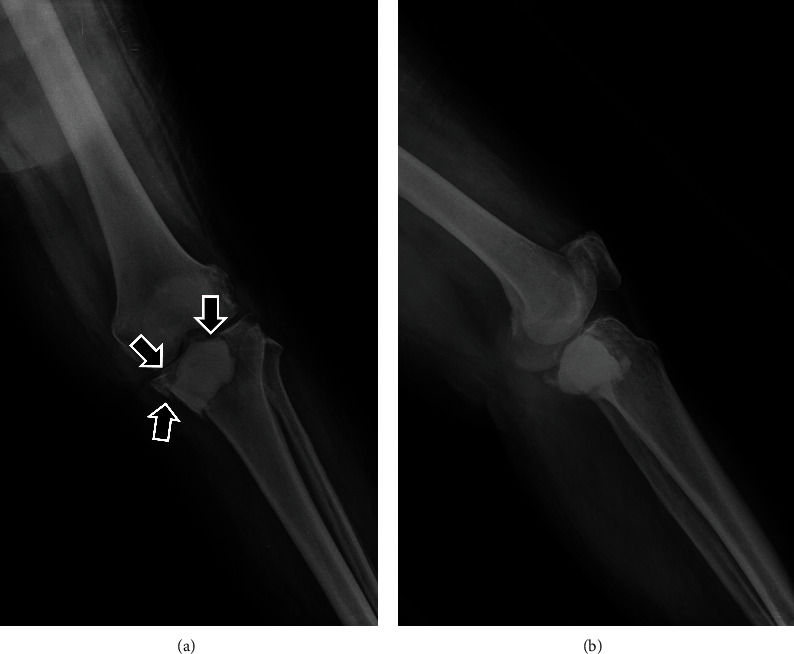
Plain radiograph ((a) anteroposterior, (b) lateral) at 14 months postoperatively showing the pathological proximal tibia fracture (black arrows). The PMMA bone cement is still contained.
